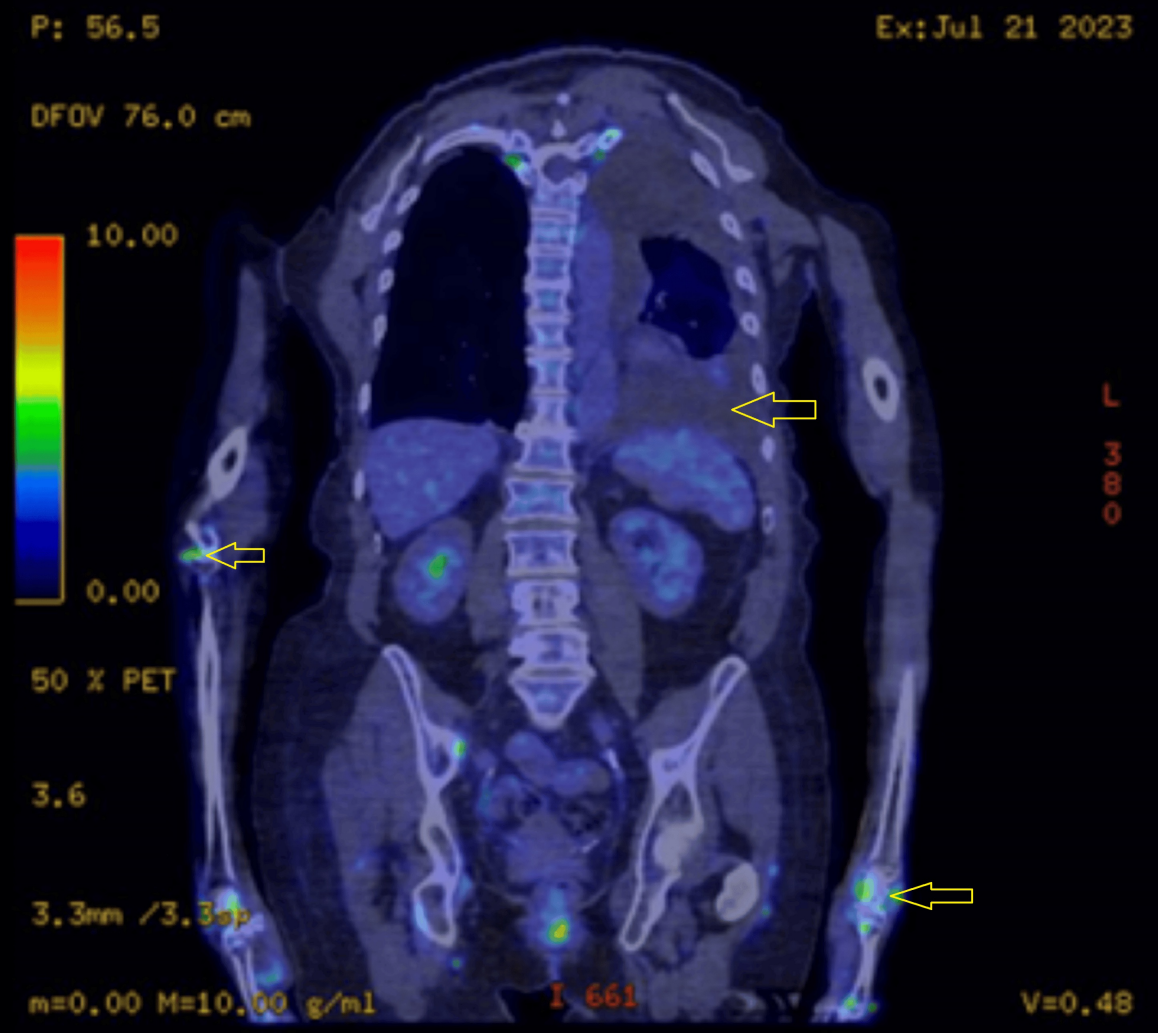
FDG PET-CT scan (coronal view) showing avid uptake in the joints with sparing of the spine and hips, and a large left pleural effusion with associated lung collapse.
